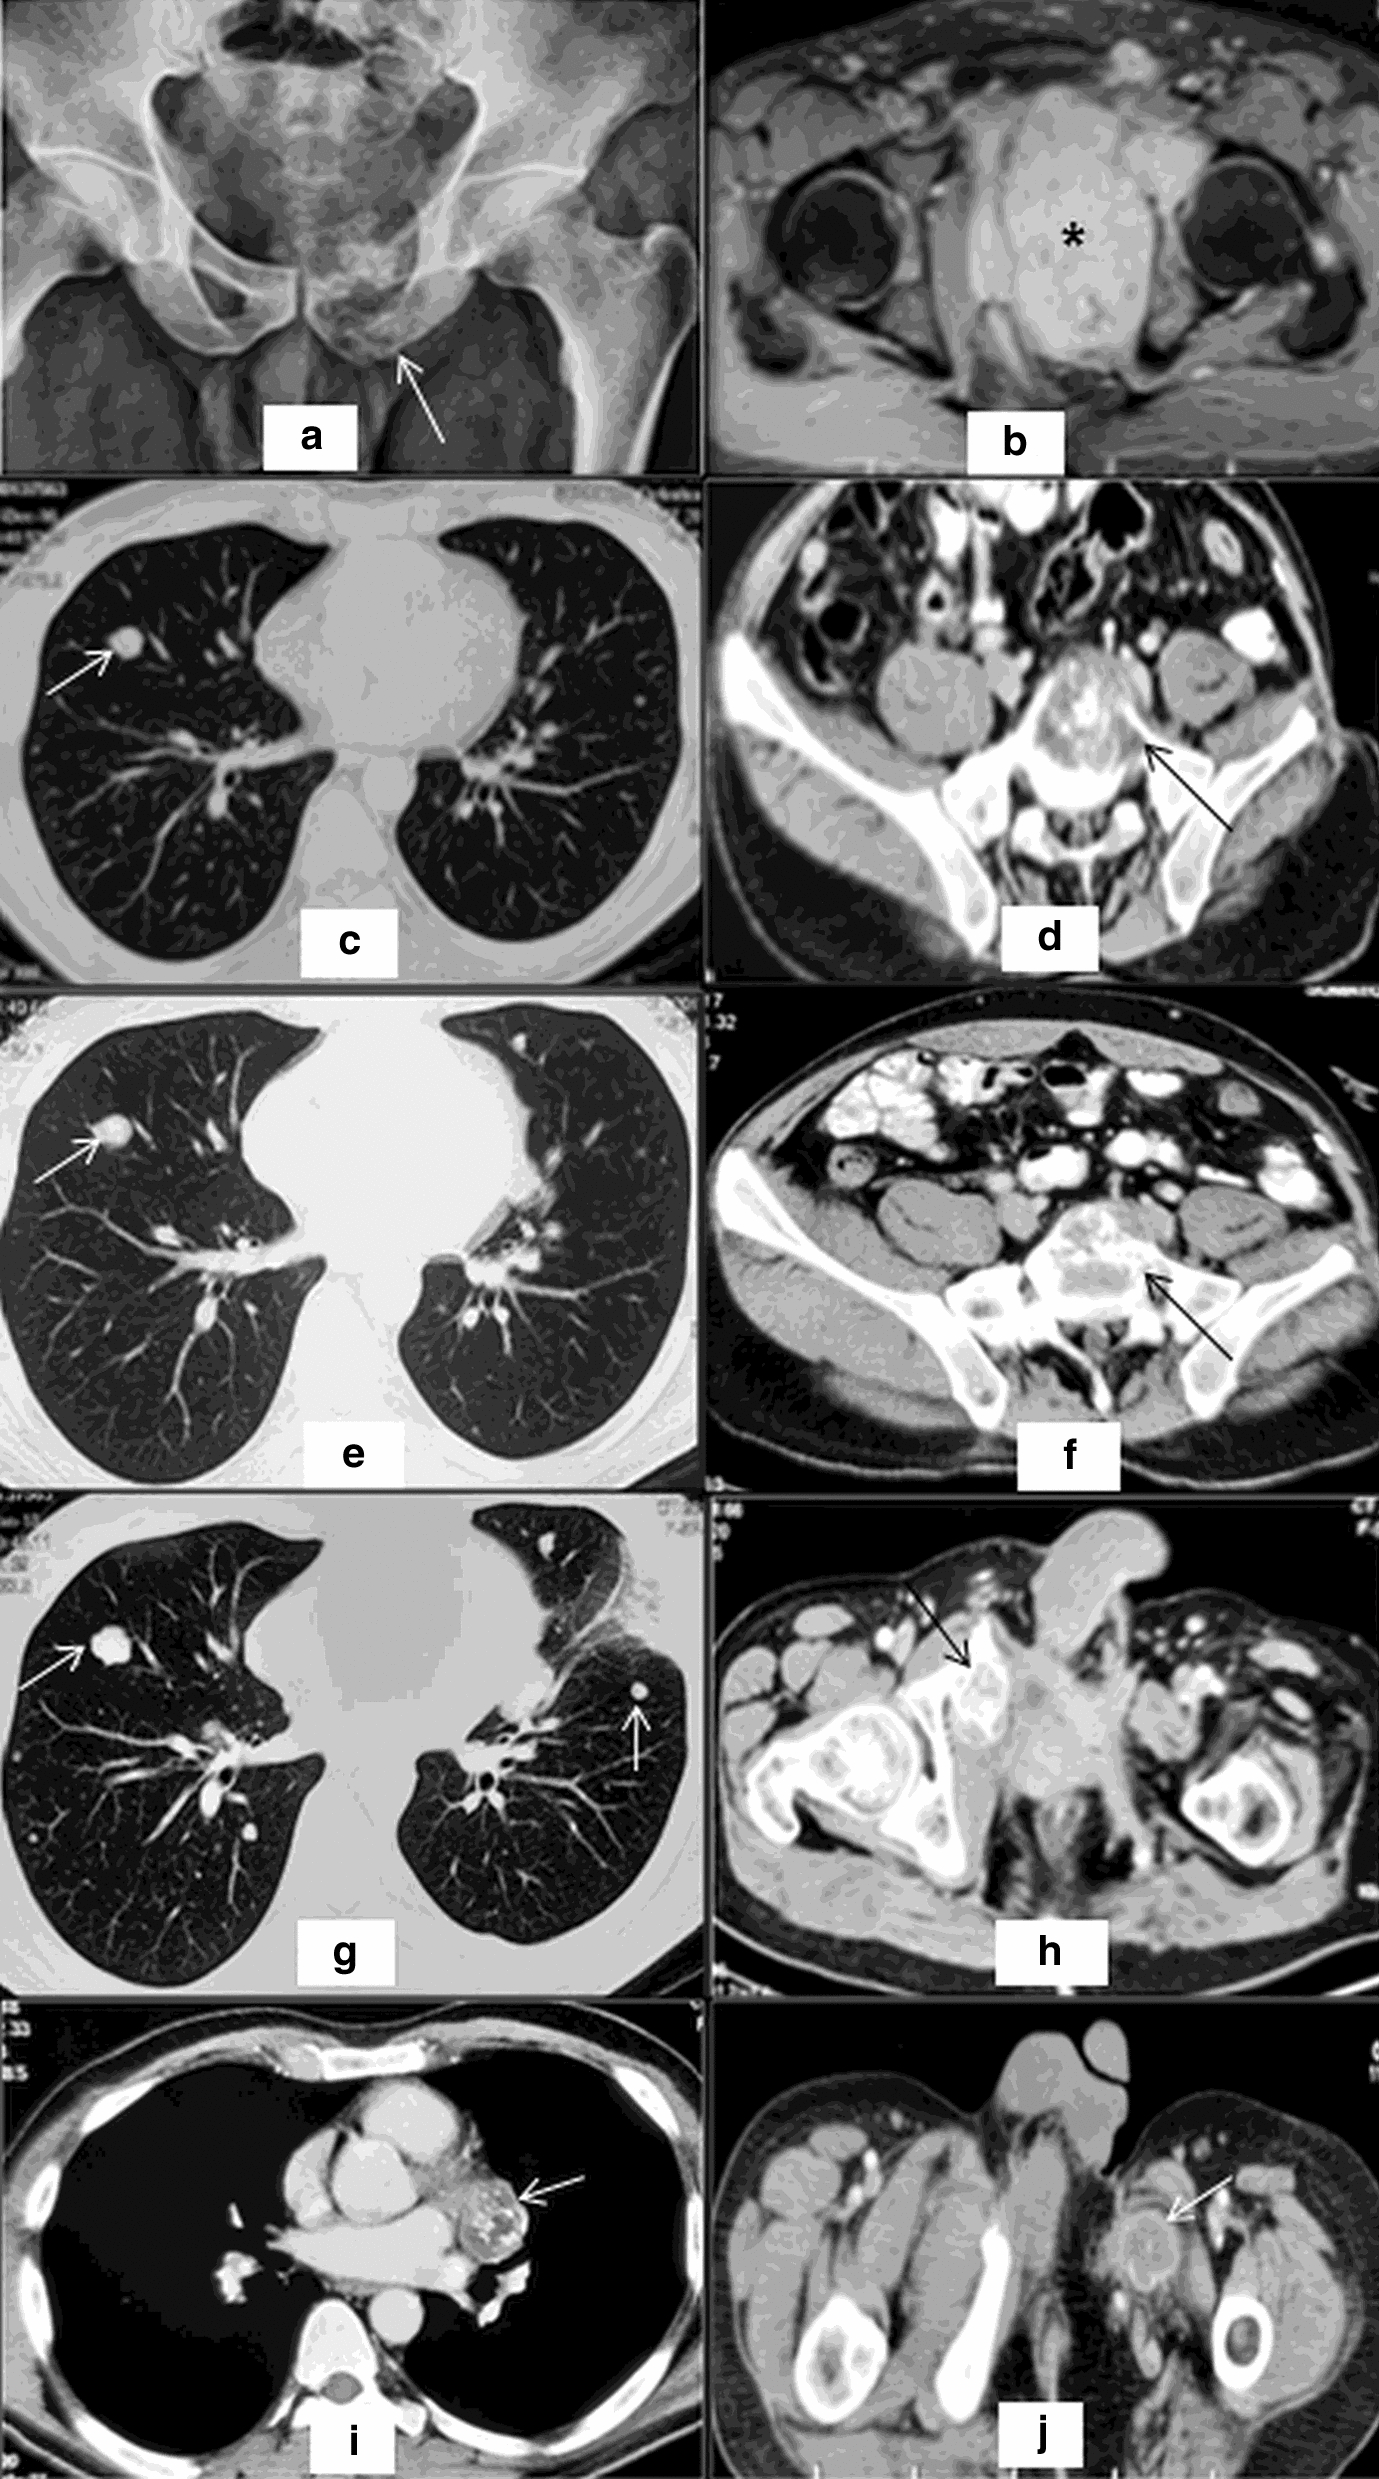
Serial imaging of the patient with pelvic chondrosarcoma. Baseline Radiograph of 2014 a showed permeative lytic destruction of left ischium with associated soft tissue component showing chondroid pattern of matrix mineralization. Corroborative MRI b showed similar findings with hyperintense mass (Asterisk) displacing the pelvic structures, not extending to hip joint. In December 2016, patient developed lung and bone metastases (arrow in c, d) after which patient was put on Trabectedin. These lesions remained stable on Trabectedin till December 2017 (e, f). CT images of January 2018 showed progression in form of appearance of new lesions in lung and bone (arrows in g, h). The disease progressed further with appearance of new lung lesions in August 2018 (arrow in i) and at local site in October 2018 (arrow in j)
